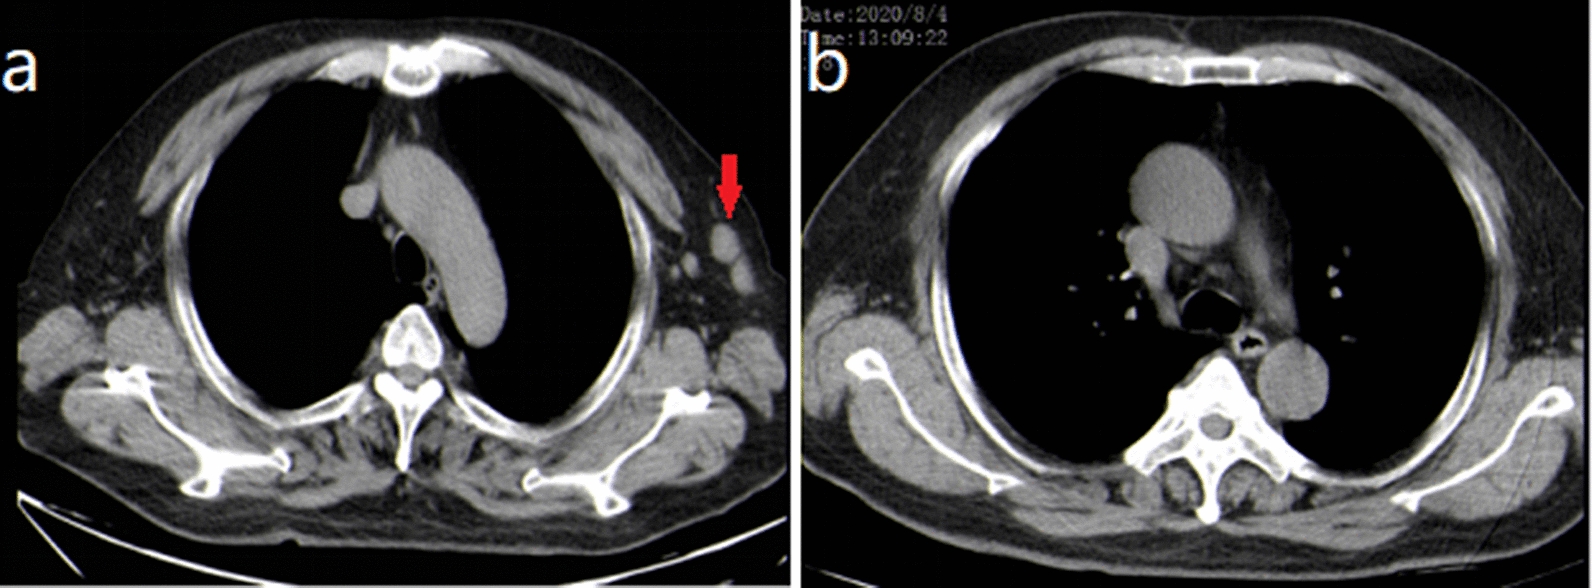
Chest CT scan: a Several enlarged lymph nodes could be seen in the left armpit in December 2018 (red arrow). b No enlarged lymph nodes found in the left armpit in August 2020
